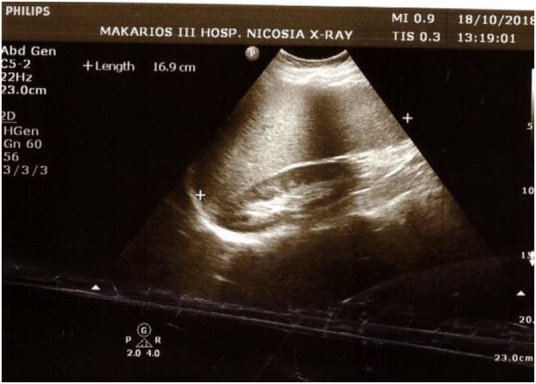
Ultrasound image of the liver and right kidney
